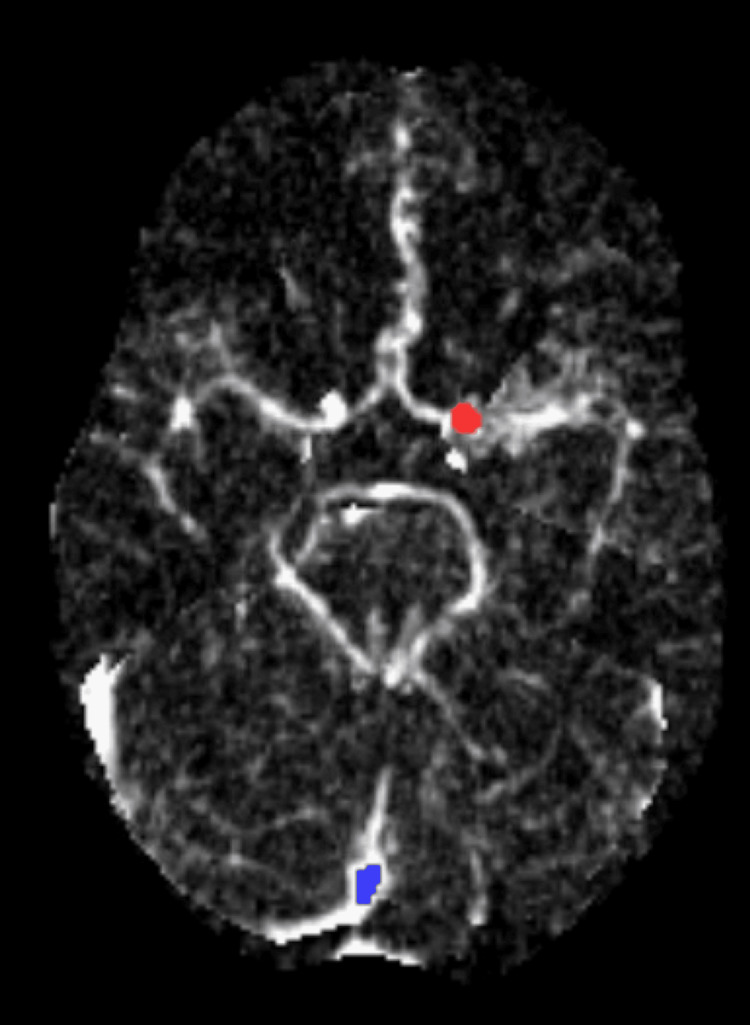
Normal CT Perfusion Normal CT perfusion with the red and blue dots showing the contrast phases performed for a neuroperfusion study. CT: Computed Tomography
